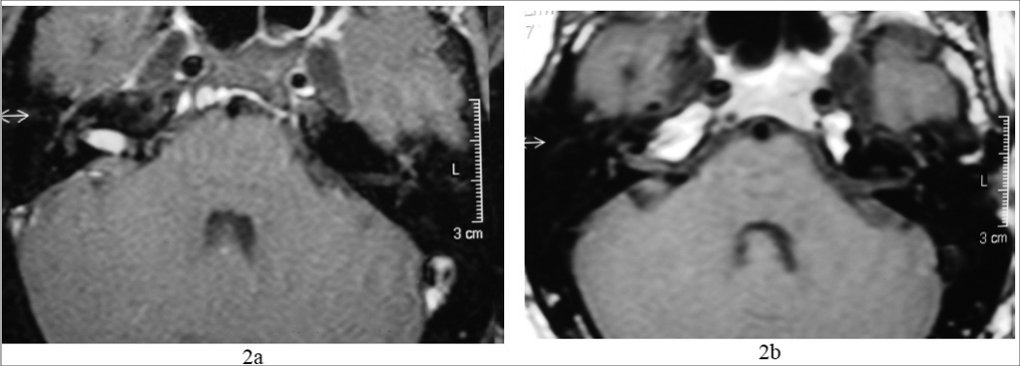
2a) MRI with gadolinium at the time of diagnosis; 2b) 4 years later, notice tumor involution.
